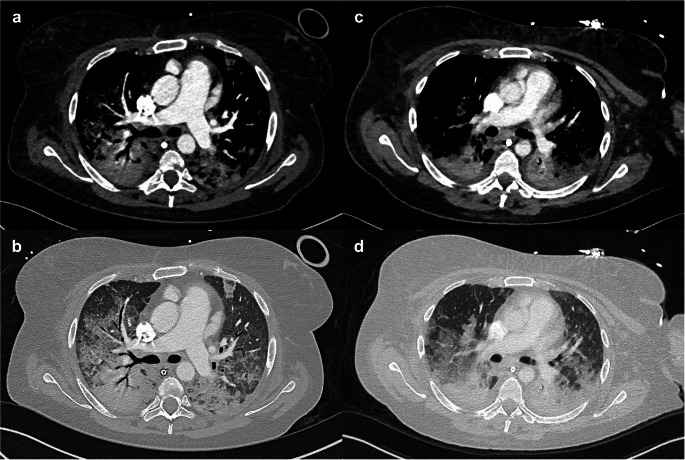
Contrast-enhanced chest Photon-counting detector CT (a, b) and Energy-integrating detector CT (c, d) obtained 7 days apart in the same female patient in her 40 s with ARDS and pneumonia
